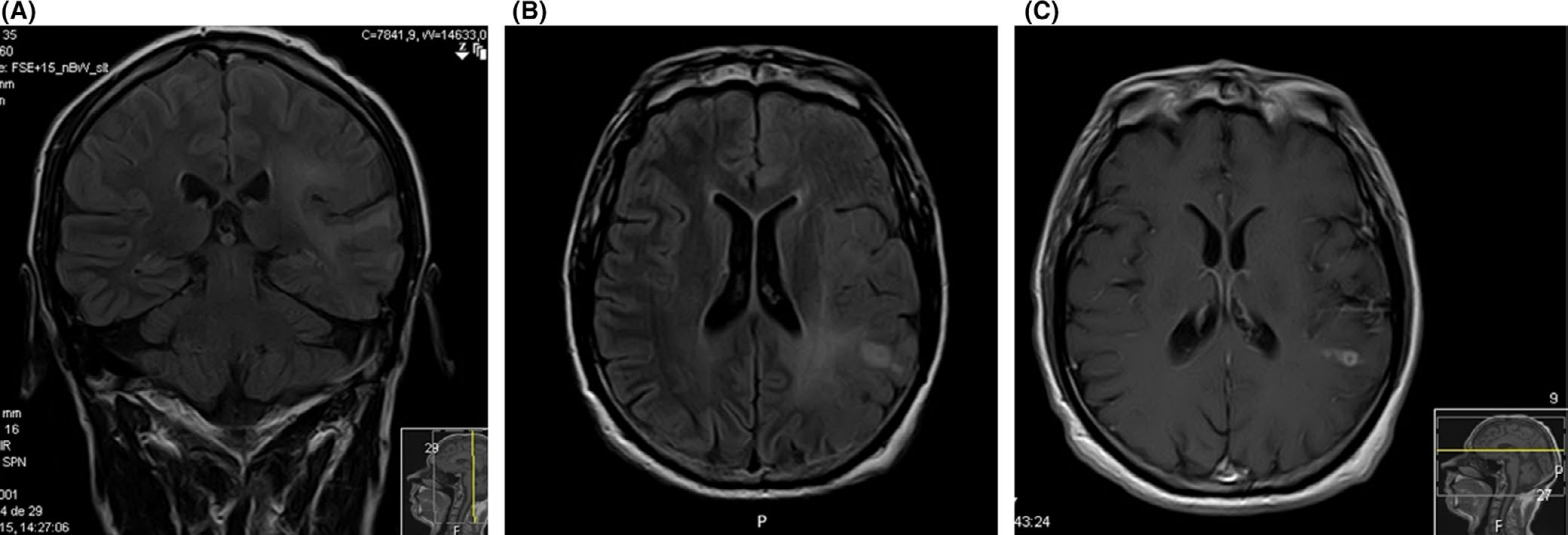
(A) Coronal flair, (B) axial flair, and (C) axial T1 + gad brain MRI sequences showing a left temporo‐insulo‐parietal glioblastoma
